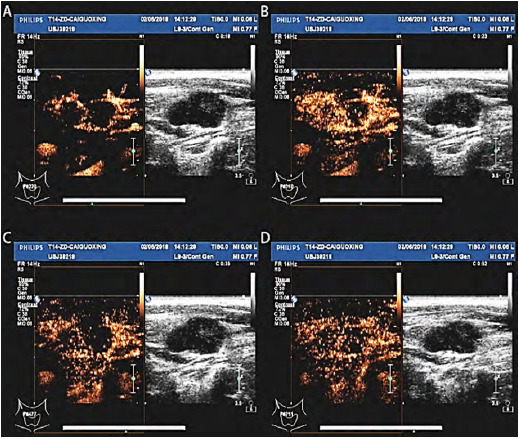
A to D: The capsule began to strengthen 16 seconds after the mass injection of ultrasound contrast agent. Enhancement peak after 23s with lymph nodes showing a centripetal pulsatile enhancement and absence of visible interior enhancement area. Clear lymph node outline at 35s and at 52s.
